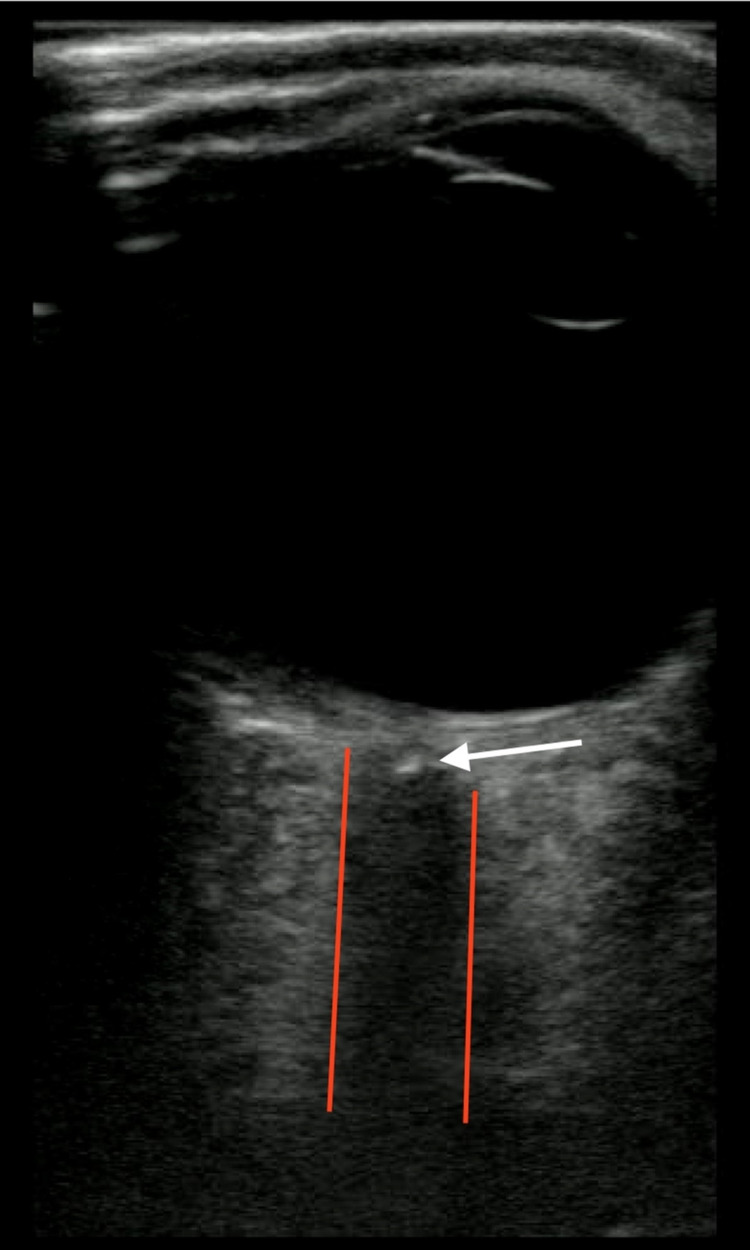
Ocular point-of-care ultrasound showing "spot sign".
